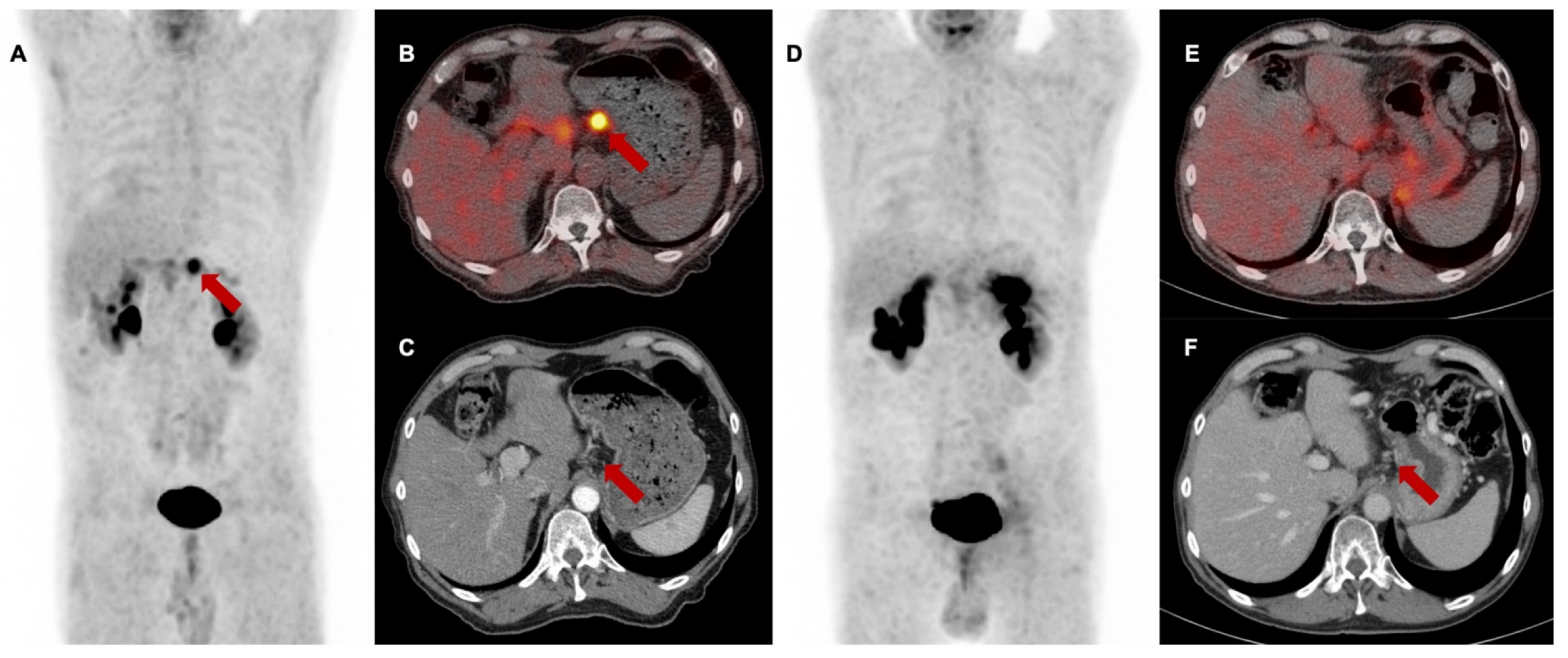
[^68^Ga]Ga-FAPI-46 PET/CT of a patient with locally recurrent pancreatic adenocarcinoma before (Baseline, A-C) and 3 months after (first follow-up D-F) treatment with chemoradiotherapy (Patient #7). A and D maximum intensity projections, B and E transaxial fusion of PET/CT of the upper abdomen, C and F transaxial contrast-enhanced CT scan of the upper abdomen. Red arrows (A-C) mark intense FAPI uptake of small-volume tumor tissue at the minor gastric margin. The red arrow in F marks stable tumor tissue without measurable FAPI signaling post-treatment. SUV_max_ at 1^st^ follow-up was 8,2 / SUV_mean_ was 5,0 and at 2^nd^ follow-up 1,7 / 1,4, respectively. In the absence of the detection of vital tumor remnants in the [^68^Ga]Ga-FAPI-46 PET/CT, complete remission of the disease was assumed. In this case, the significant decrease of the FAPI uptake in the 1^st^ follow-up scan was associated with local tumor control within the next 6 months after therapy. Patient #7 showed local progression in the 3^rd^ follow-up scan 9 months post CRT.
